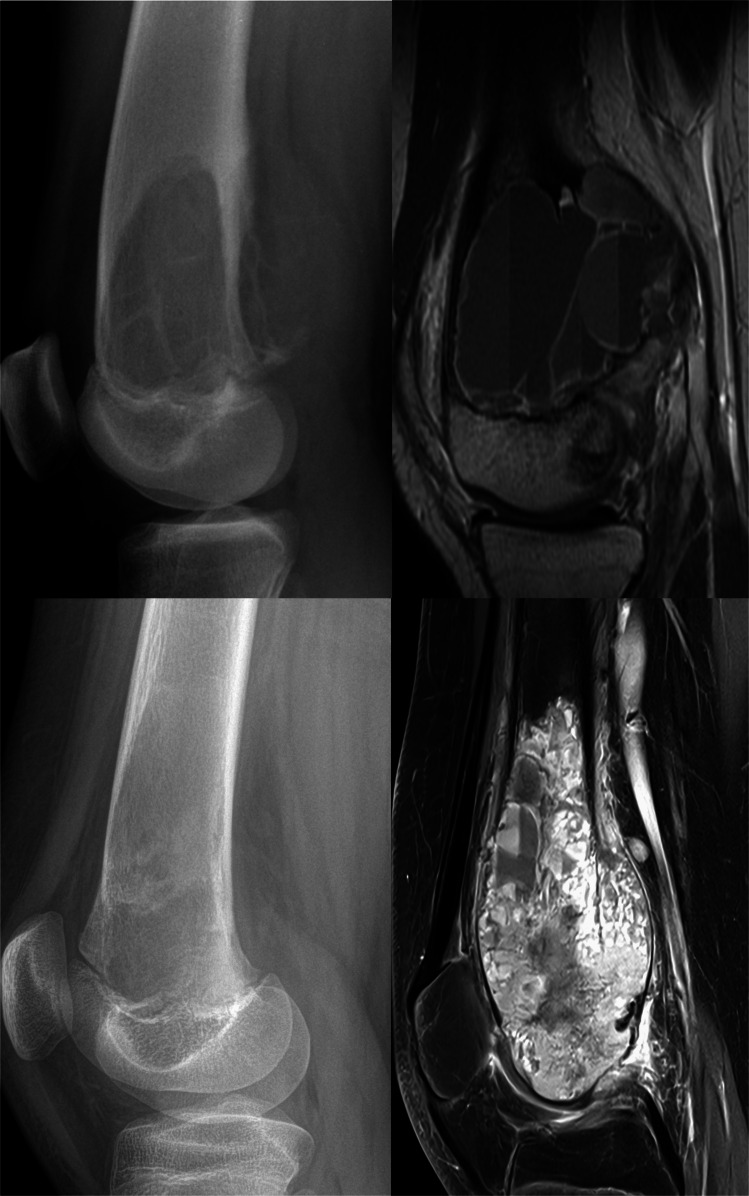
Comparison between ABC and telangiectatic osteosarcoma. Fourteen-year-old female with aneurysmal bone cyst (top images). Lateral radiograph (top left) of the knee demonstrates a well-defined expansile lytic lesion within the distal femoral metaphysis with a significant posterior component resulting in an attenuated cortex which is imperceptible posteriorly. Sagittal T1-weighted image post-contrast (top right) reveals a multiloculated lesion comprising entirely of multiple fluid–fluid levels with peripheral and septal enhancement, and importantly, no significant enhancing soft tissue component. Twelve-year-old female with telangiectatic osteosarcoma (bottom images). Lateral radiograph (bottom left) demonstrates an ill-defined distal femoral metadiaphyseal lytic lesion with regions of faint lesional sclerosis. Sagittal T1FS post-contrast image (bottom right) shows a complex tumour with multiloculated components, fluid–fluid levels in addition to prominent areas of nodular enhancing solid lesional tissue
